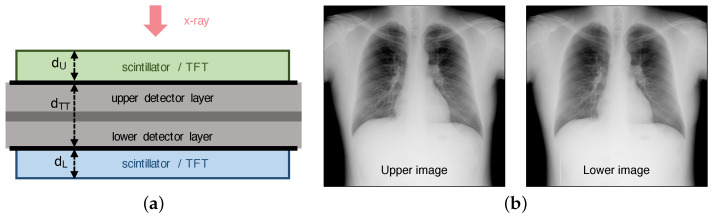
Structure of the dual-layer flat-panel detector (DFD) and acquired images (DRTECH Co., Ltd., Seongnam, Republic of Korea, www.drtech.com). (a) Two flat-panel detector layers are attached as close as possible. Conventionally, the upper and lower layers can have the same direction as the incident X-ray. In this example, the lower layer is inverted. The upper layer can also be inverted [8]. $d_{\mathrm{TT}}$ is the distance between TFT layers, and $d_{U}$ and $d_{L}$ are the thicknesses of the upper and lower scintillator layers, respectively. (b) An example of chest images acquired from a DFD. The pixel pitch is 0.14 mm, and the image size is 3072×3072 pixels, where $d_{\mathrm{TT}}=1.1$ mm and $d_{U}=d_{L}=0.5$ mm.
